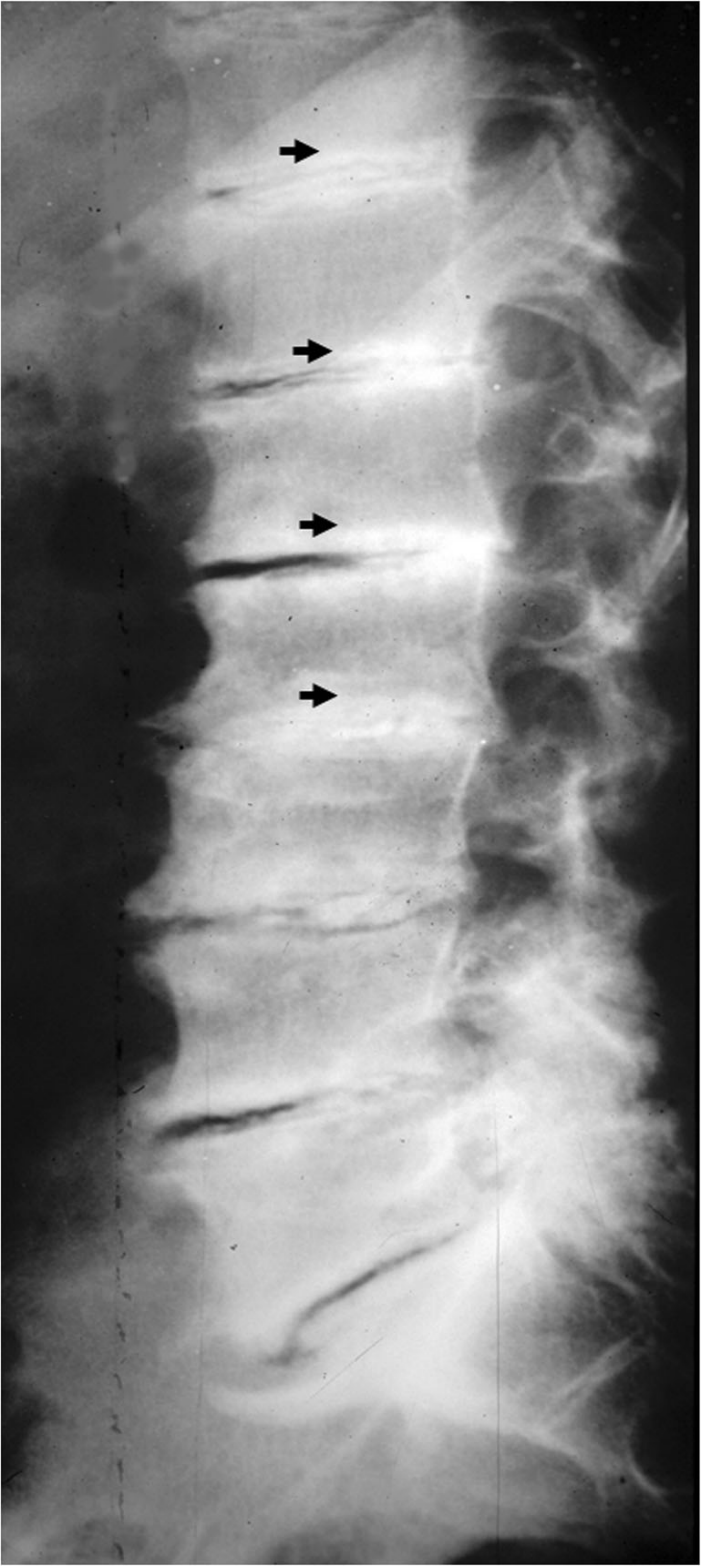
Plain x-ray film of the spine. Diffuse osteosclerosis of the spine in a patient undergoing chronic haemodialysis. Diffuse sub-endplate densities at multiple contiguous levels (*arrows*), a pattern known as “rugger-jersey spine”
